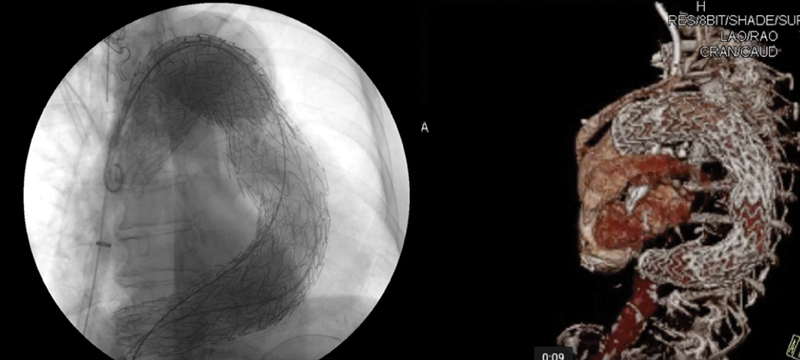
The left panel shows the intraoperative angiography with total exclusion of the aneurysm and no sign of endoleak. The right panel shows the predismissed computed tomographic angiogram with normal ascending aorta and no sign of endoleak.
